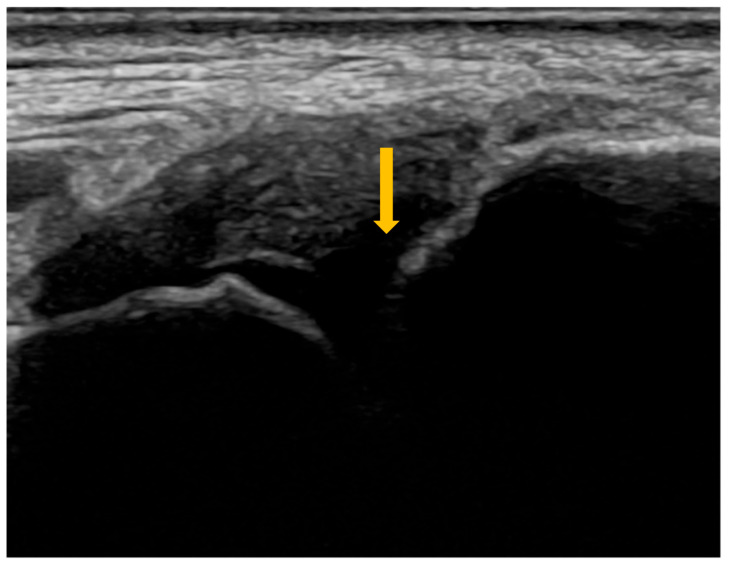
B-mode ultrasound of the right radiocarpal joint demonstrating synovial hypertrophy. Gray-scale (B-mode) ultrasound of the right radiocarpal joint shows synovial hypertrophy, appearing as thickened hypoechoic synovial tissue within the joint recess (arrow), consistent with active inflammation.
